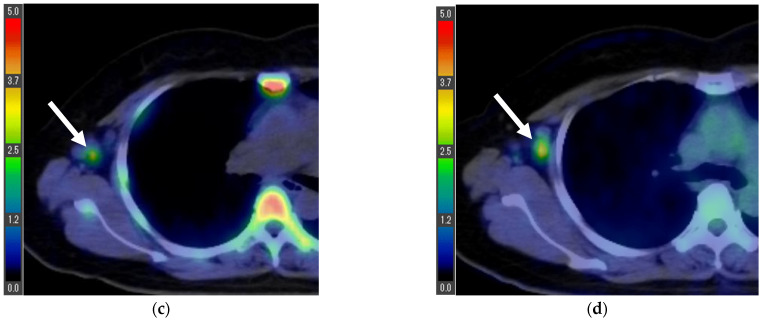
Maximum intensity projection (MIP) of ^18^F-FLT PET (a), MIP of ^18^F-FDG PET (b), axial image of ^18^F-FLT PET/CT (c), and axial image of ^18^F-FDG PET/CT (d). The axillary lymph node that showed the greatest accumulation differed between the ^18^F-FLT PET/CT and ^18^F-FDG PET/CT, with SUVmax values of 3.0 and 3.4, respectively (arrows). Patient 1 (Figure 1).
